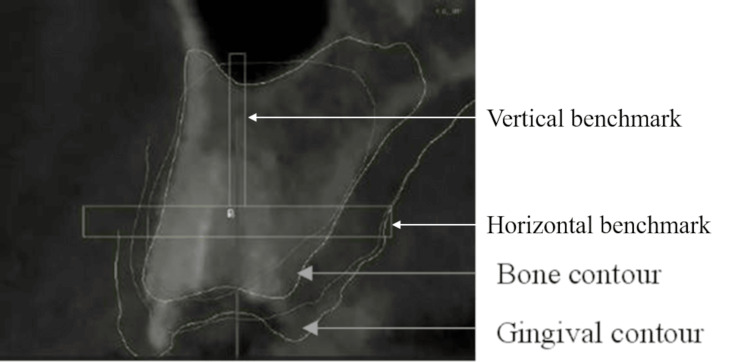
CBCT sagittal section at T0 showing four contours: bone and gingival contours at T0 (green) and T1 (orange), vertical virtual benchmark (pink), and horizontal virtual benchmark (yellow)
